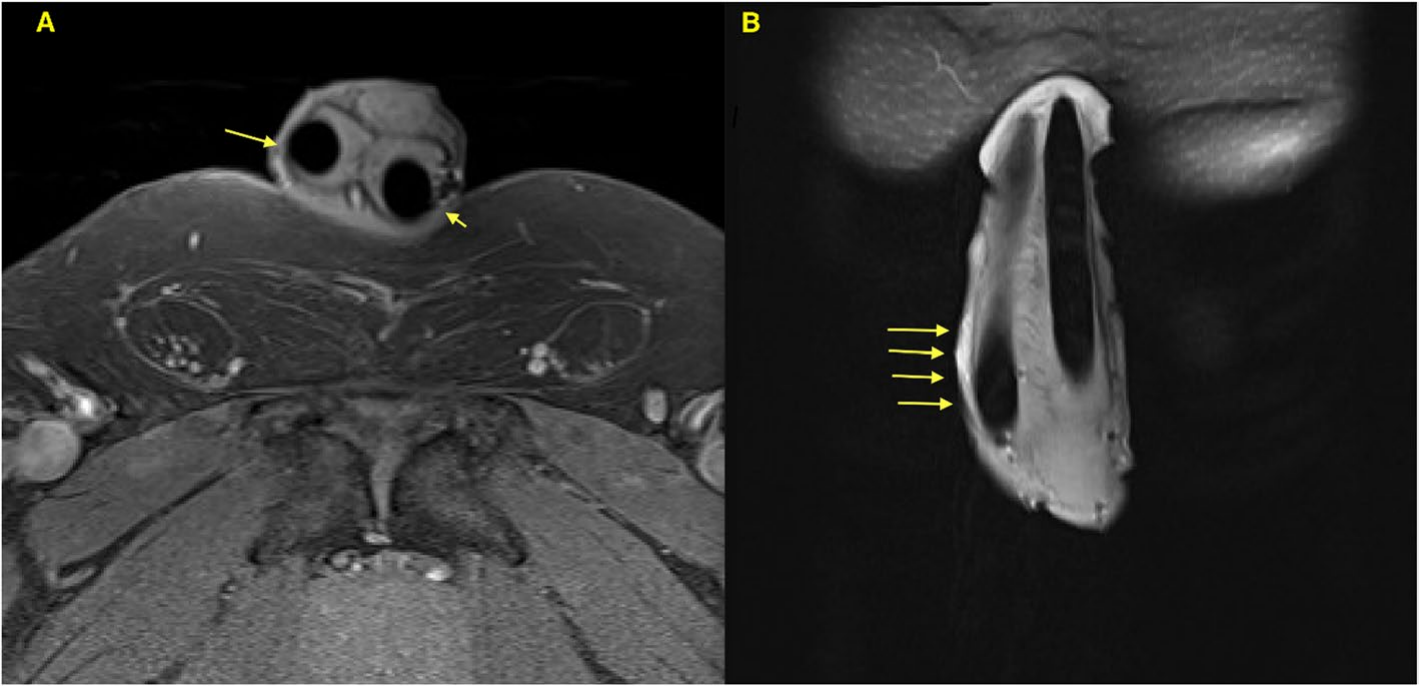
Penile magnetic resonance images of Patient 2 following initial device replacement without plication corporoplasty. A Axial view showing thin and weakened tunica albuginea bilaterally (arrows). B Coronal view highlighting the device angulation site at the right corpora cavernosa (arrows)
